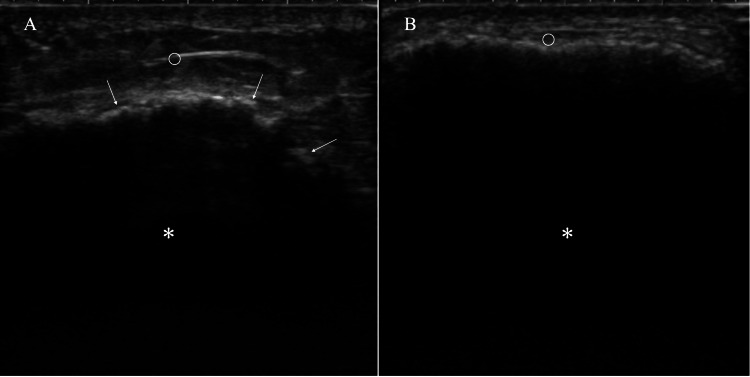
Ultrasound findings (A) Ultrasound showed subcutaneous fat approximately 1 cm thick (open circle) just above the RFA nodule (asterisk) despite the impossible observation of the internal structures of the RFA nodule due to the presence of dystrophic calcifications (arrows). (B) Ultrasound clarified the marked decrease of subcutaneous fat (open circle) just above the RFA nodule (asterisk) at the onset of cellulitis. RFA: radiofrequency ablation
